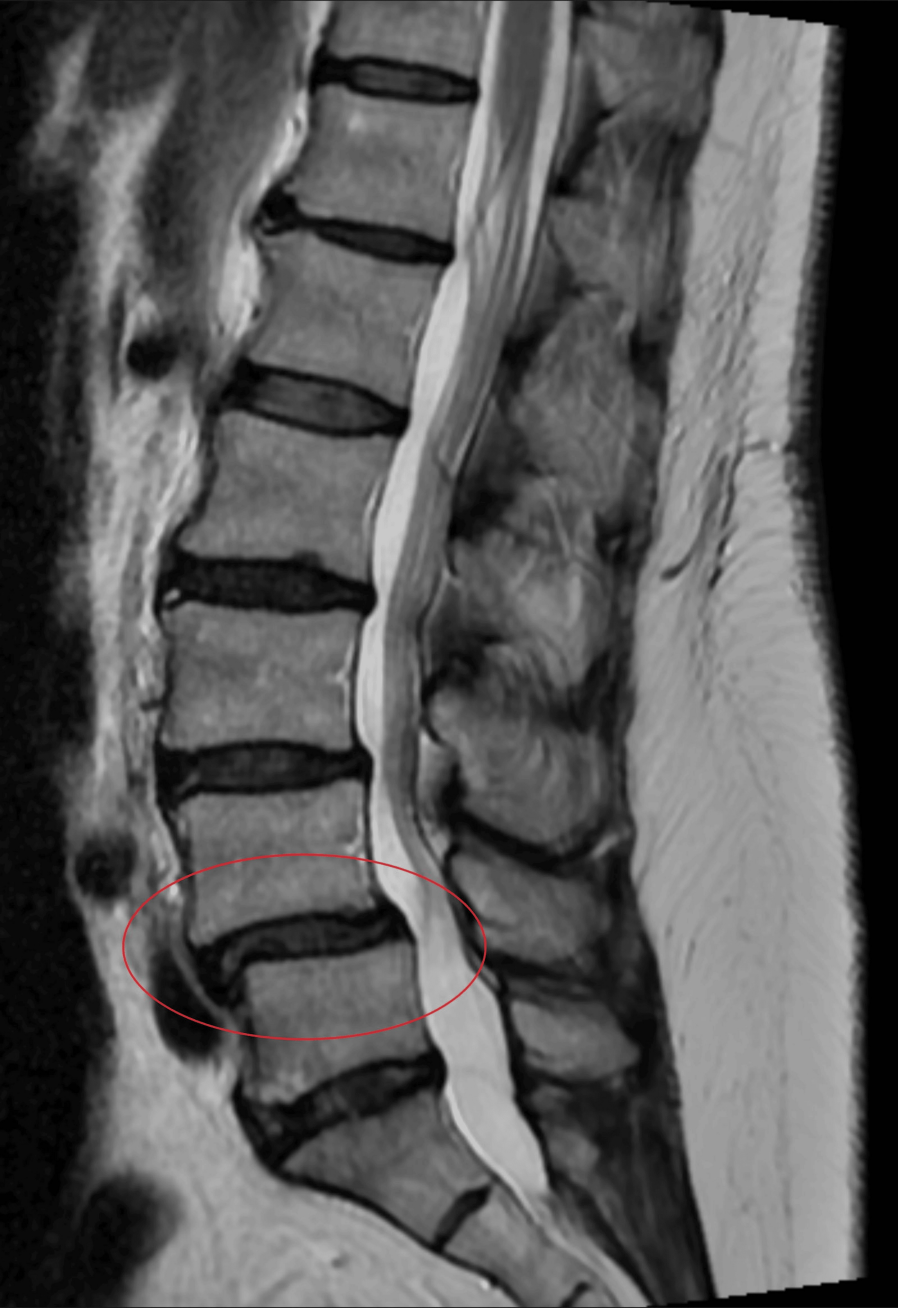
Sagittal view of lumbar MRI 10 months before surgery showing L4-L5 spondylolisthesis (circled)
